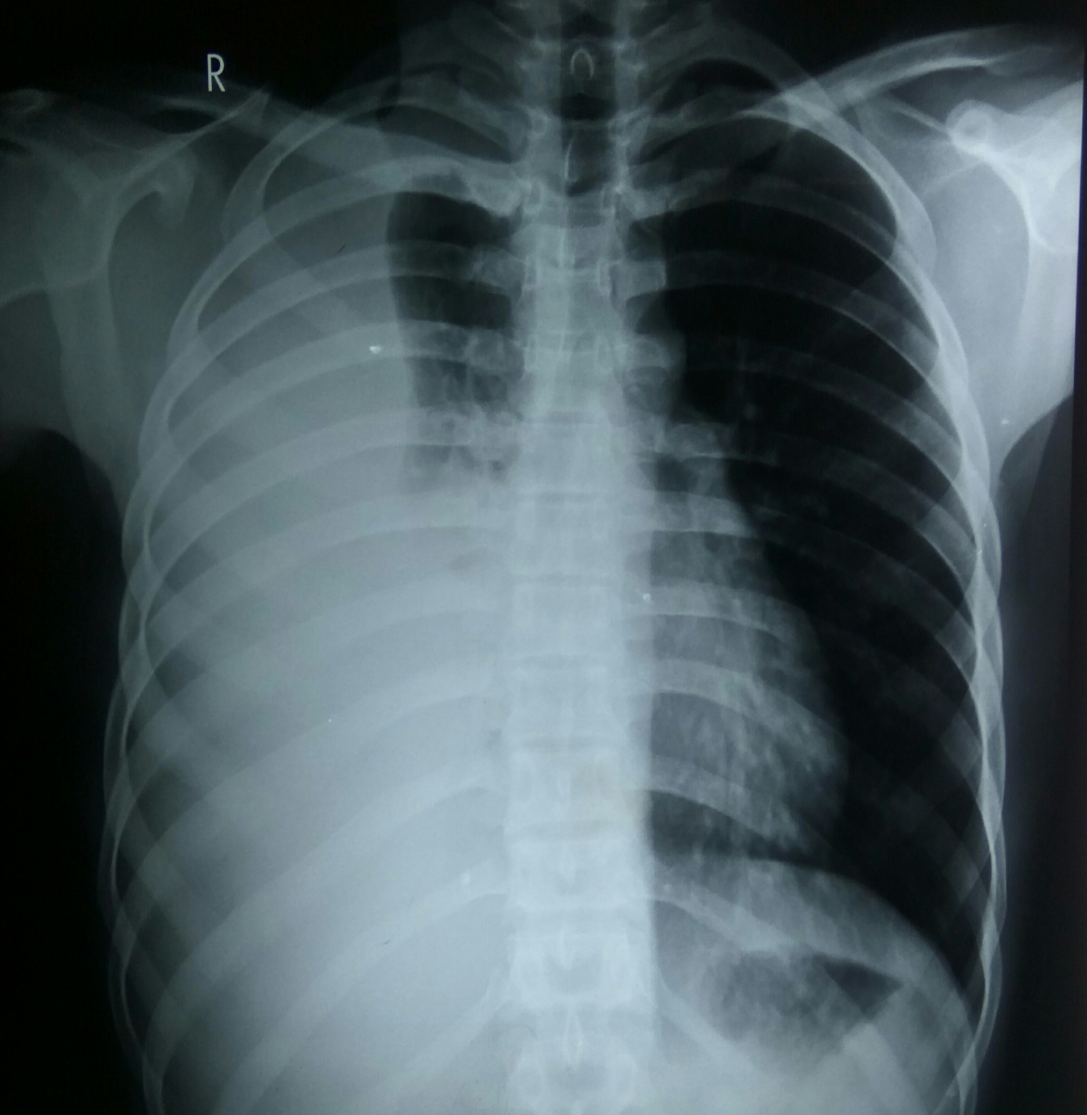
Posteroanterior erect chest X-ray of patient on initial presentation.
